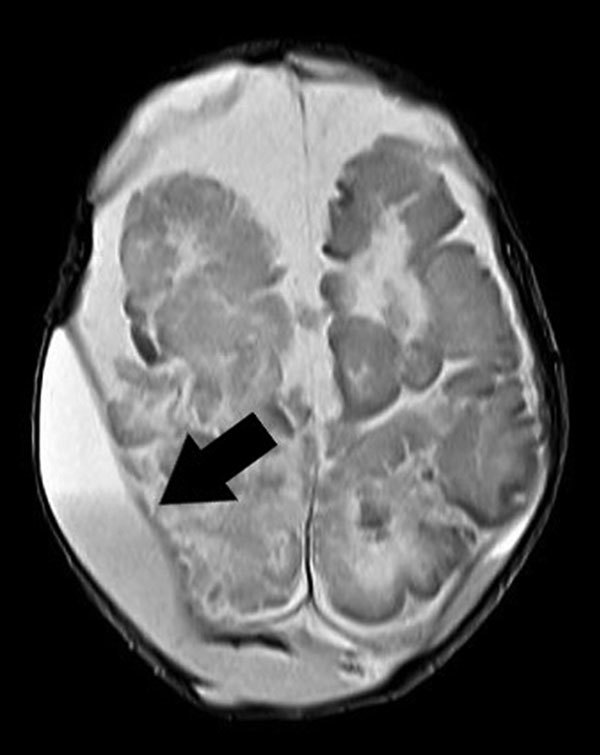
Brain nuclear magnetic resonance image of a newborn with *Cronobacter sakazakii* sequence type 494 meningitis, Brazil. Extradural collections are visible in both parietal regions. Arrow indicates the more pronounced extradural collection, measuring ≈1.8 cm, in the right parietal region.
